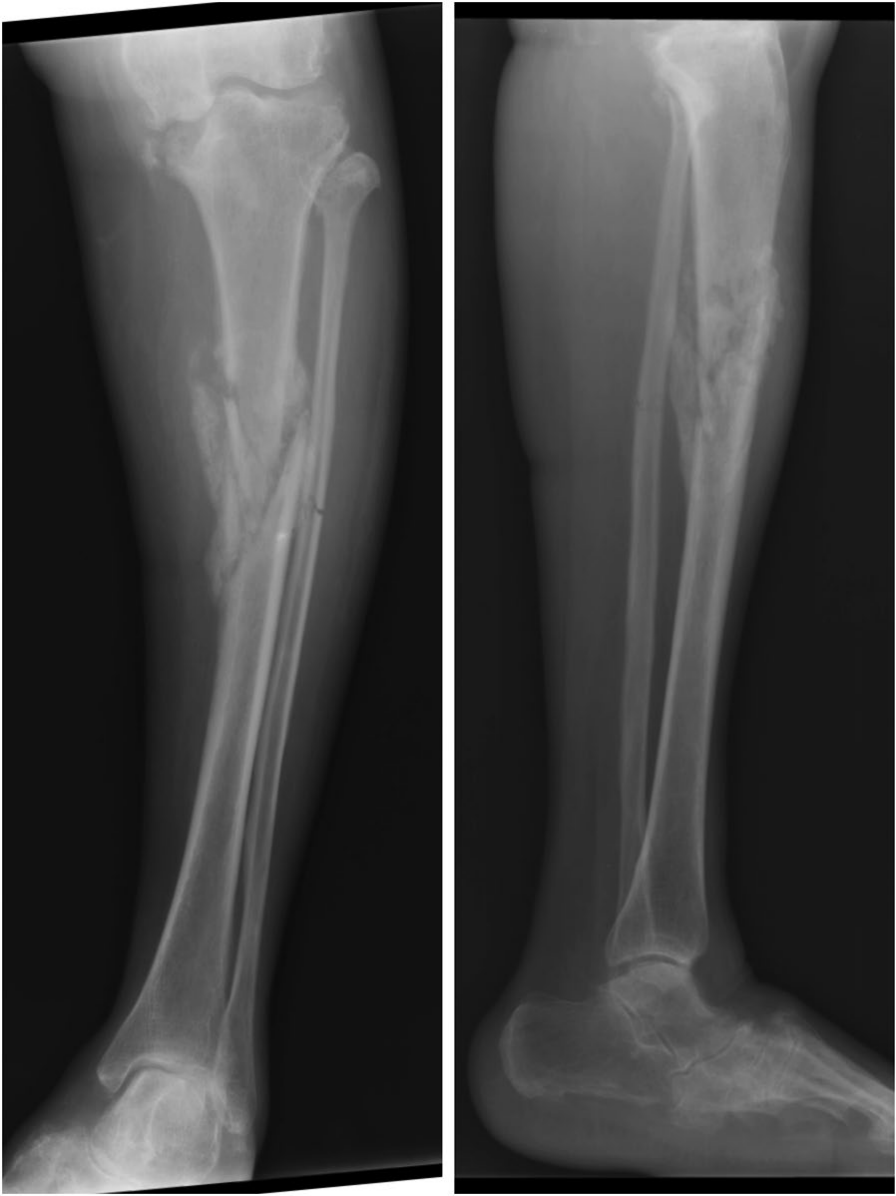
Case 1 preoperative radiographs depicting grade 4 osteoarthritis of the knee with stress fractures (both extraarticular and intraarticular). The patient had history of long standing knee pain since many years, with inability to walk since the last 4 months. The tibial alignment appears to be in severe varus (> 20°)
